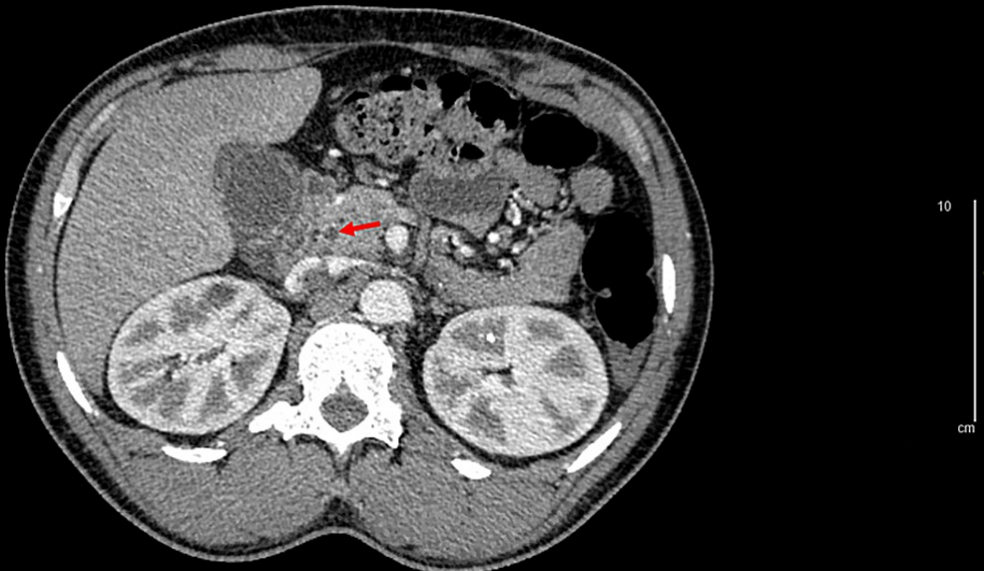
CT scan on admission showing 0.6 cm dilation of the common bile duct (red arrow) at 1.42x magnification.
